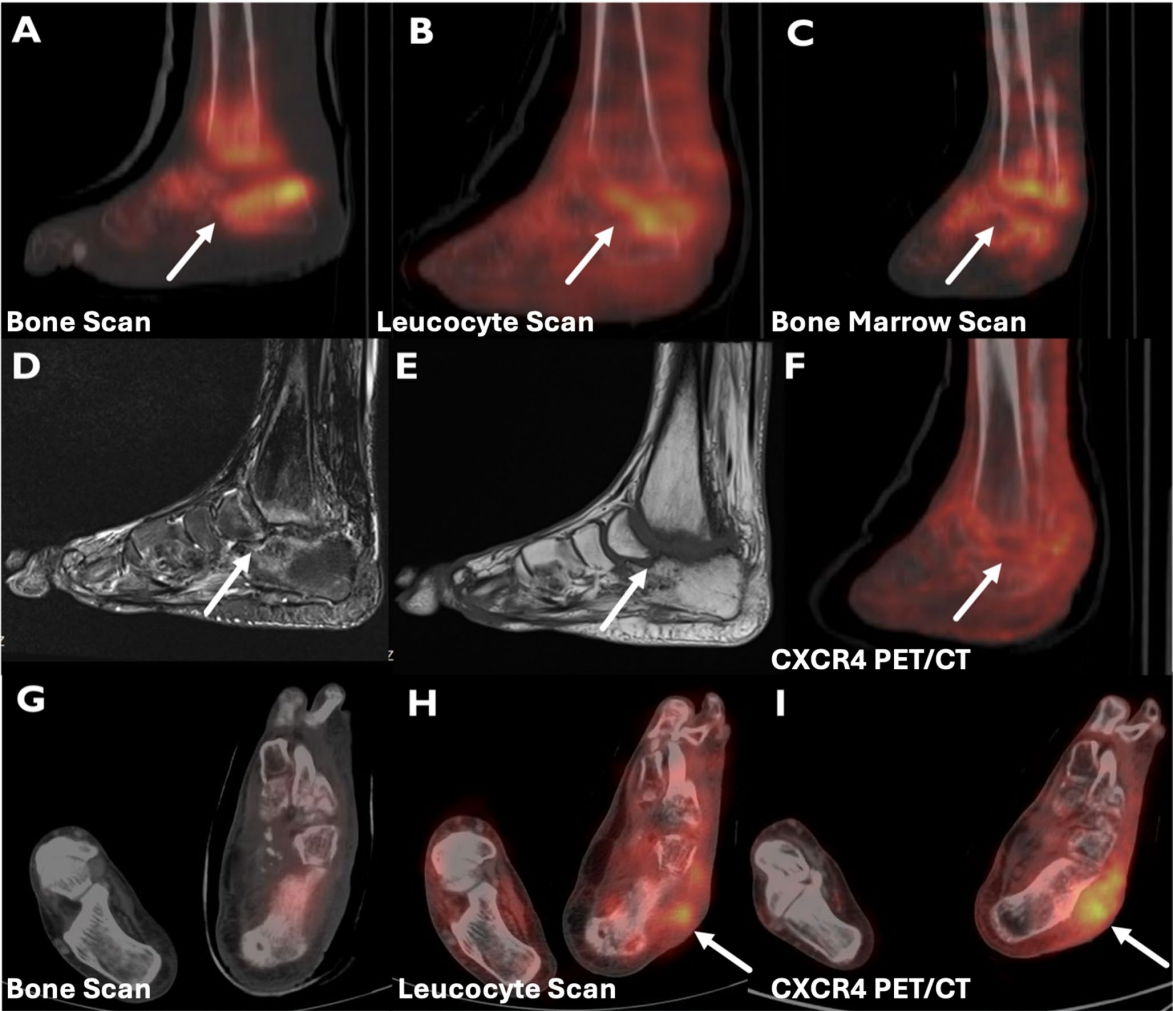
35-year-old male with diabetes and left foot chronic (6 years) neuropathic arthropathy. BS (A), LLS (B), BMS (C) increased uptake in tibiotalar and tarsometatarsal joints consistent with CNA; MRI T2 hyperintensity/T1 hypointensity (D, E). CXCR4 PET mild heterogeneous uptake in the CNA region (F). Lateral talar soft tissue uptake on LLS (H) and CXCR4 PET (I) but not on BS (G), suggesting soft tissue infection; culture: MRCoNS. No OM on follow-up; patient underwent arthrodesis for CNA.
